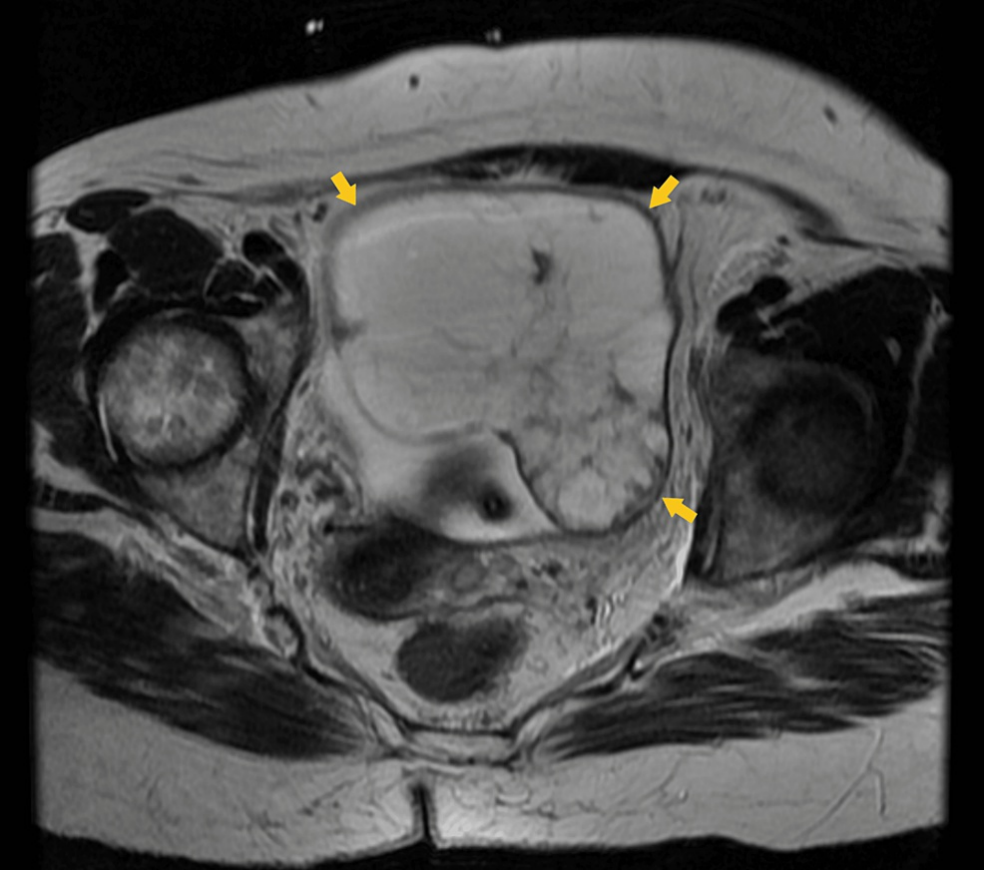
Magnetic resonance imaging of a large ovarian tumor. The axial T2-weighted image demonstrates a left complex cystic mass with solid areas and dimensions of 10.9x8.4 cm (arrows).
